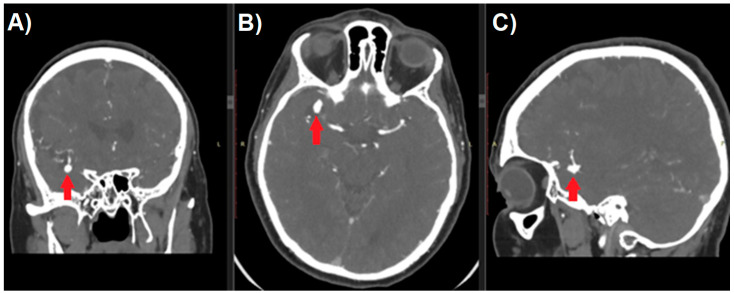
Multi-planar reconstruction of Computed Tomography Angiography study of patient with cerebral aneurysm (red arrow). Digital Imaging and Communications in Medicine (DICOM) image in (A) coronal, (B) axial, and (C) sagittal plane.
